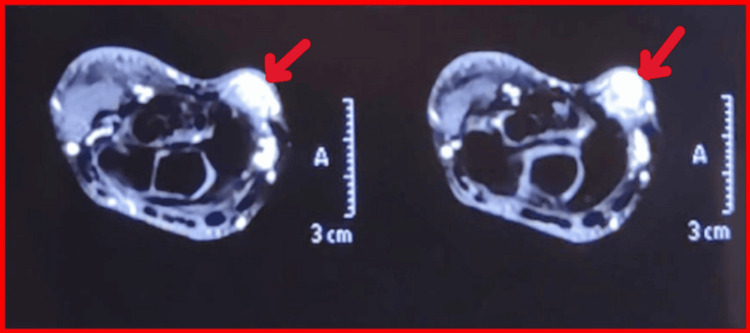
Axial T2-weighted MRI sections showing the lesion The TGCT is T2 hyperintense and is marked by a red arrow. TGCT: tenosynovial giant cell tumor; MRI: magnetic resonance imaging
